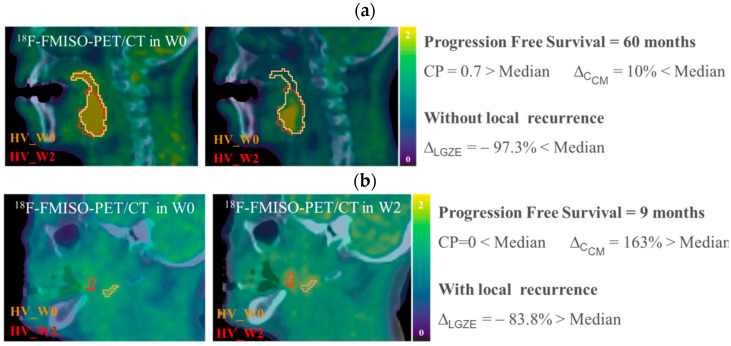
Example of ^18^F-FMISO-PET/CT images, HSV contours and values of CP, ∆_C_CM__ and ∆_LGZE_, for (a) P01, patient without local recurrence and longer progression free survival, and for (b) P20 patient with local recurrence and shorter progression free survival. All ^18^F-FMISO-PET images were represented with a scale of (0, 2) g/mL and superimposed to the corresponding CT.
